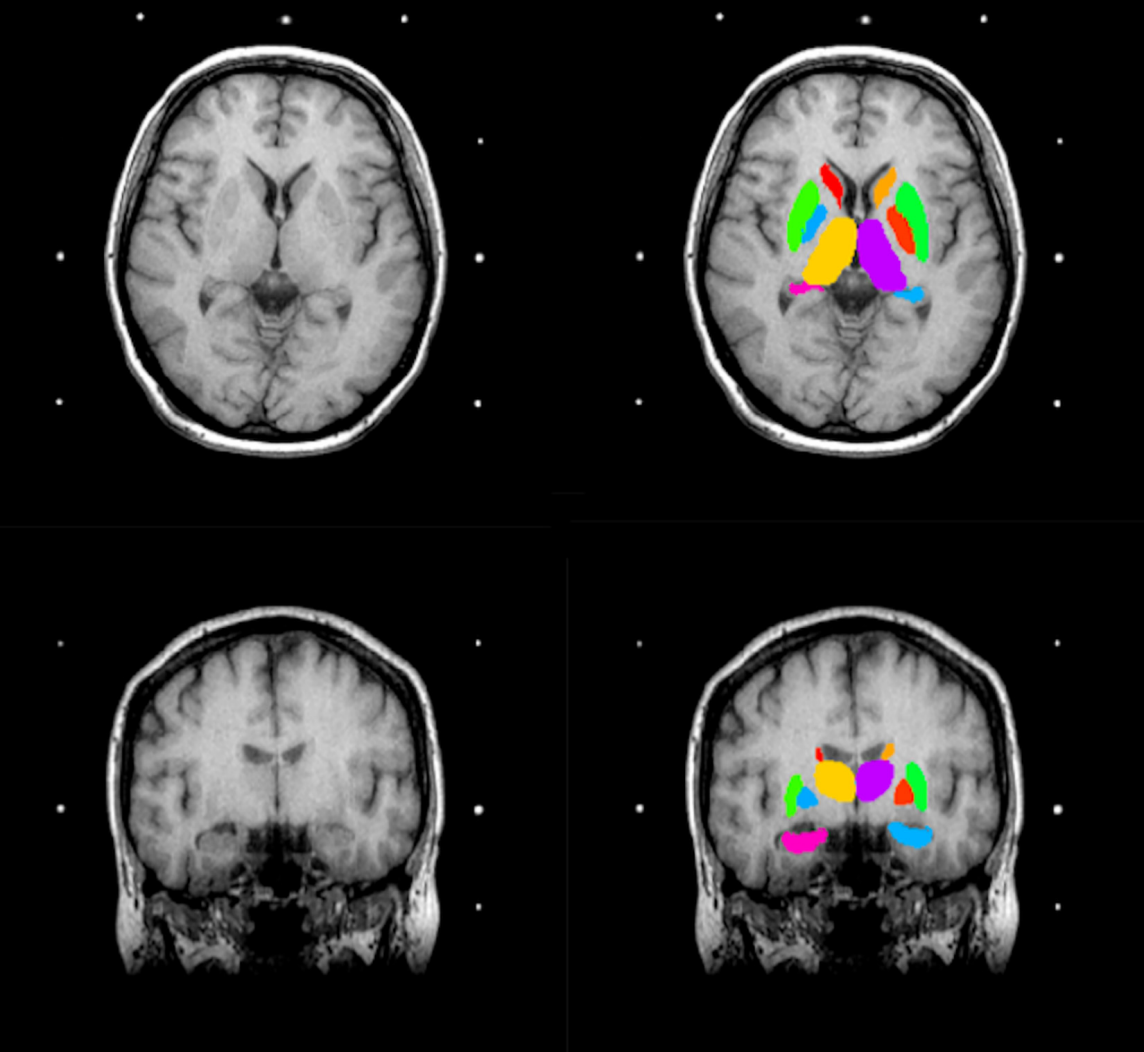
Brain MR images. Axial (top) and coronal (bottom) Brain MR image before (left) and after (right) application of FIRST. The colours correspond to the segmented sub-cortical structures: pink (left hippocampus), light blue (right hippocampus), orange (left thalamus) and purple (right thalamus).
